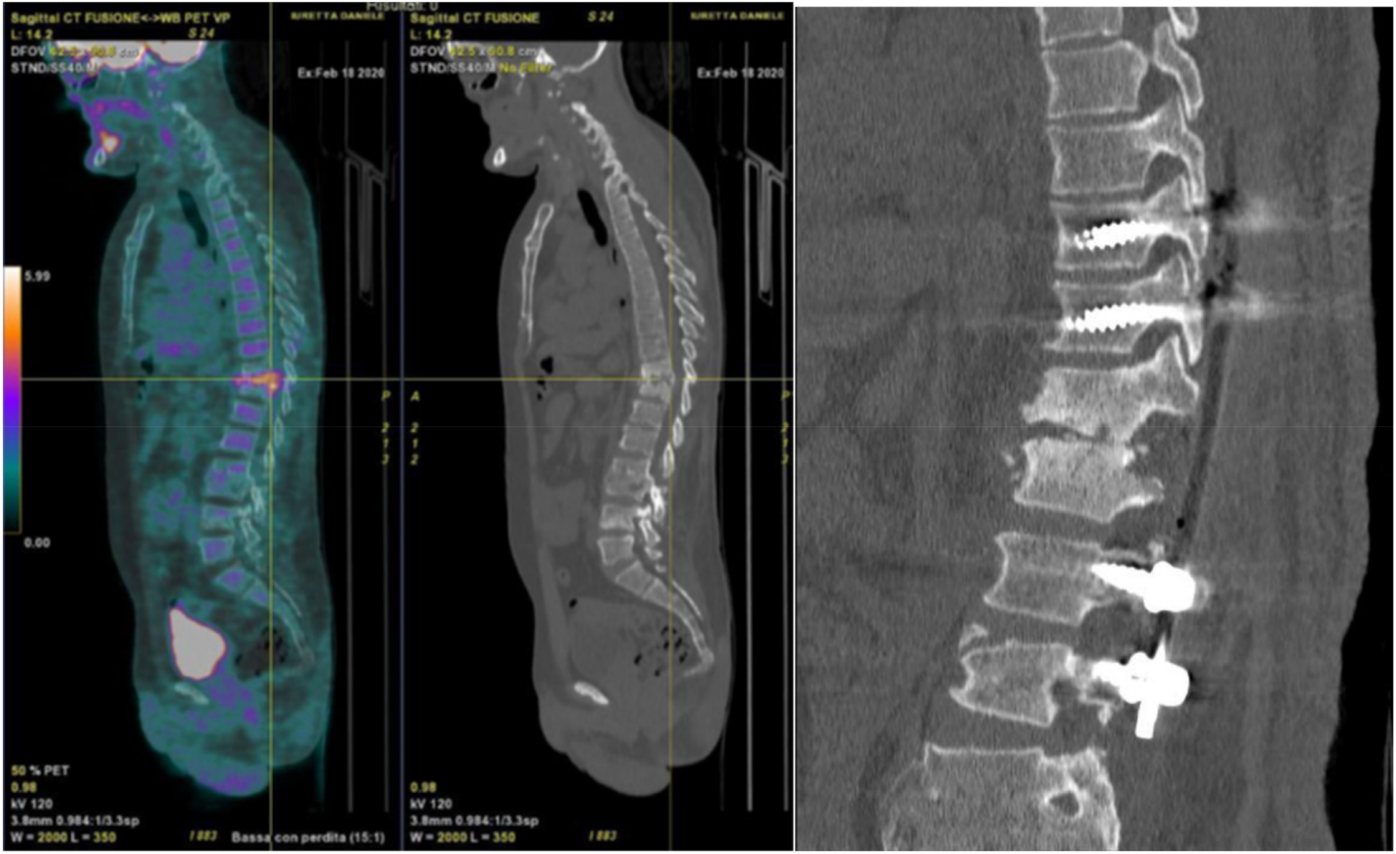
Left, PET-CT with a hyper caption of 18-FDG at the level of the infection. Right, postoperative CT showing decompression and posterior arthrodesis T10–L1 with silver-coated instrumentation.
